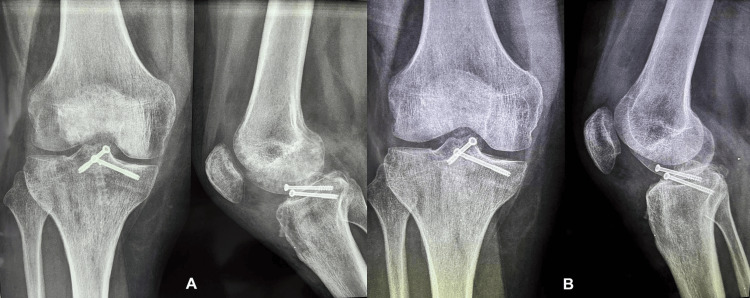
Postoperative (anteroposterior and lateral views) radiographs of the patient's ACL-PCL reconstructed knee. (A) Postoperative X-rays at three months. (B) Postoperative X-rays at six months. Radiological fracture healing can be observed not only at six months but also at three months post-op. ACL: anterior cruciate ligament; PCL: posterior cruciate ligament
